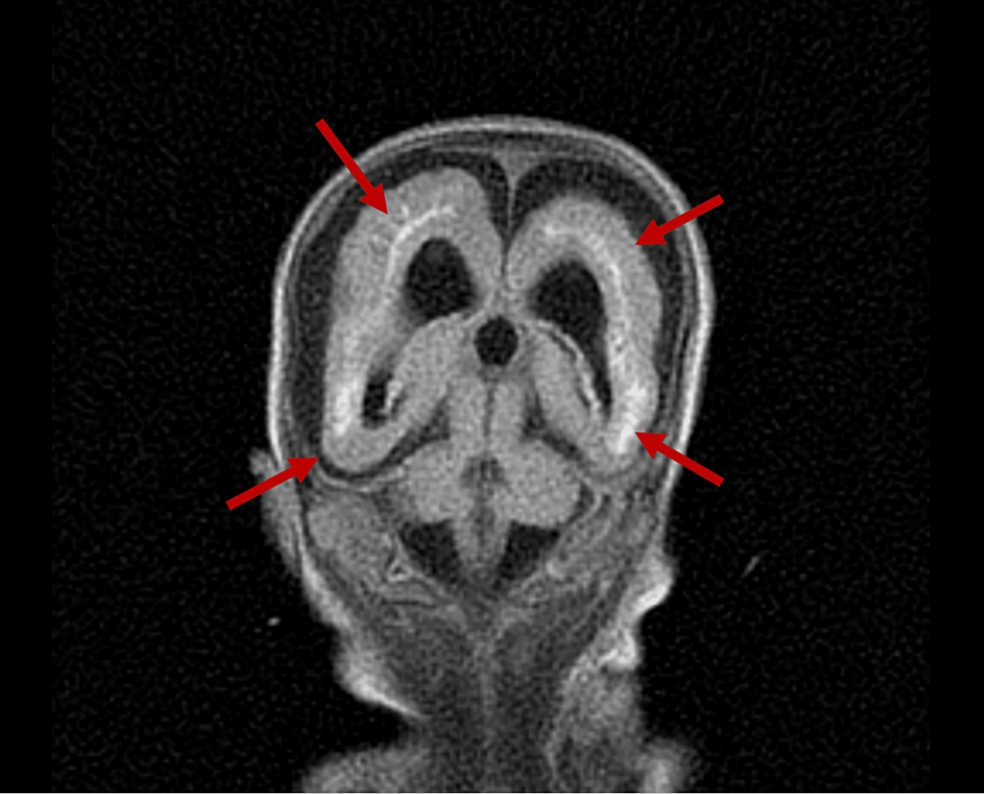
Coronal plane of brain MRI at day of life 31, displaying generalized brain calcifications (red arrows)
